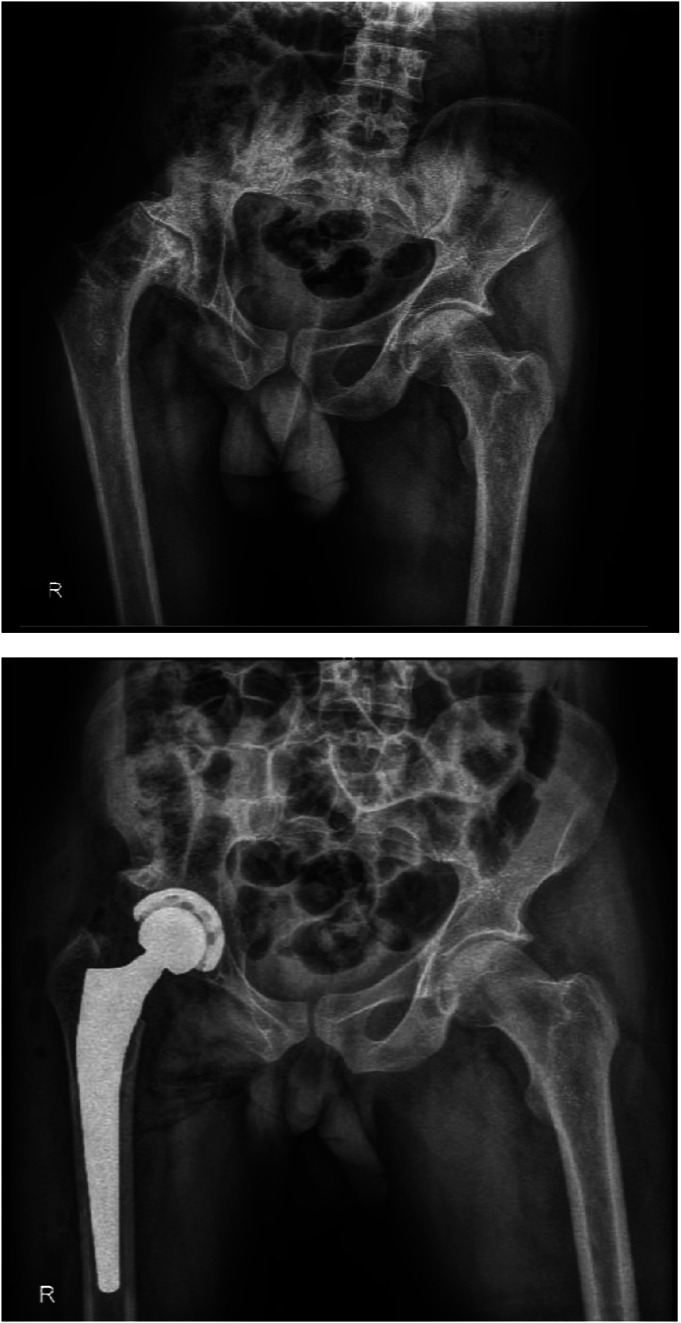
Preoperative and postoperative radiographs of a 28-year-old male patient with avascular necrosis.
